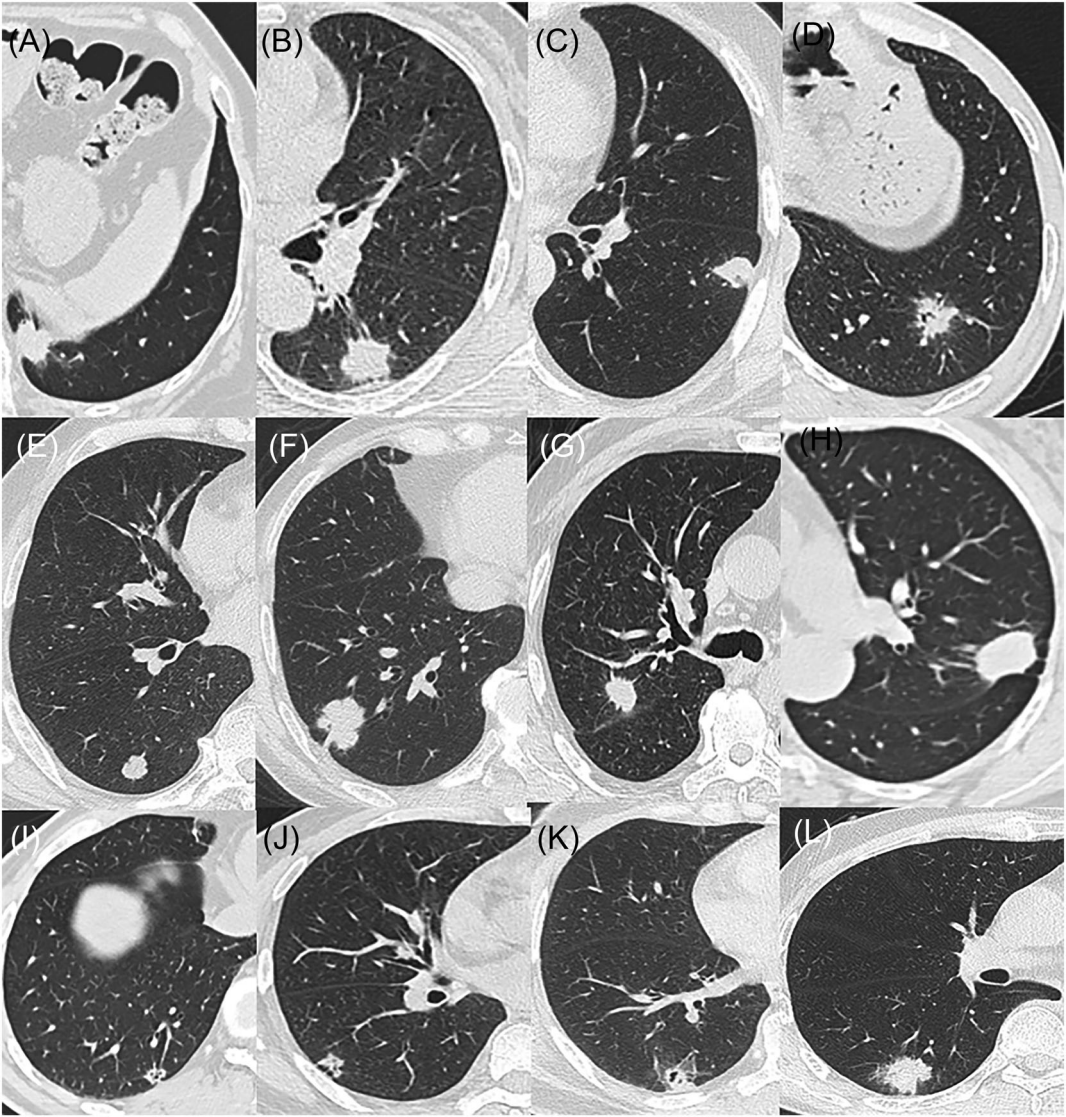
CT manifestations of pulmonary mucinous adenocarcinoma with a solitary nodule/mass with varying sizes. (A) The nodule was located in the posterior basal segment of the left lower lobe. (B) The nodule was located in the dorsal segment of the left lower lobe. (C) The nodule was located in the anteromedial basal segment of the left lower lobe. (D) The nodule was located in the basal segment of the left lower lobe. (E) The nodule was located in the posterior basal segment of the right lower lobe. (F) The nodule was located in the basal segment of the right lower lobe. (G) The nodule was located in the posterior segment of the right upper lobe. (H) The nodule was located in the tip‐posterior segment of the left upper lobe. (I) The nodule was located in the basal segment of the lower lobe of the right lung. (J) The nodule was located in the anterior basal segment of the right lower lobe. (K) The nodule was located in the posterior basal segment of the right lower lobe. (L) The nodule was located in the dorsal segment of the right lower lobe.
